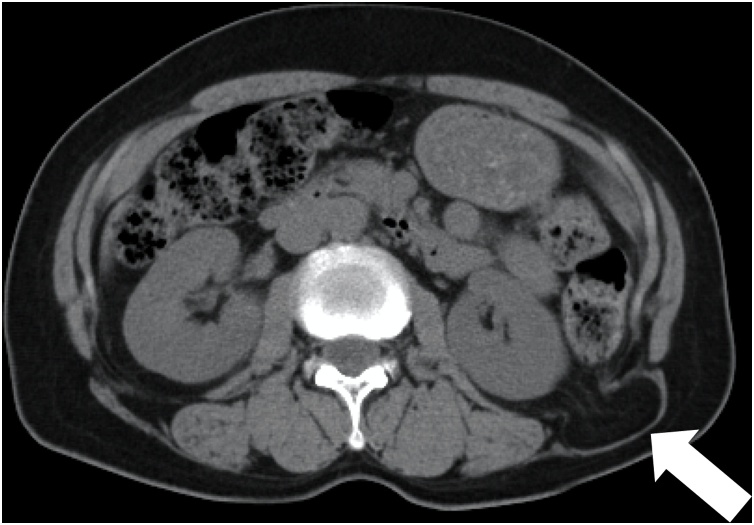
Computed tomography revealed the left superior lumbar hernia (white arrow). The size of hernia orifice was 20 × 14 mm.
